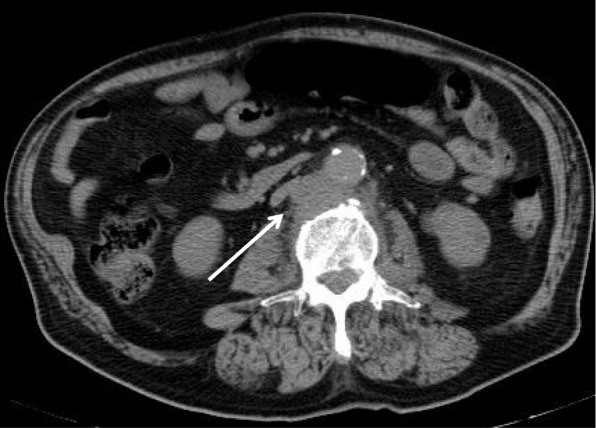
Plain abdominal computed tomography scan of our patient. The mycotic abdominal aortic aneurysm is shown by an arrow
